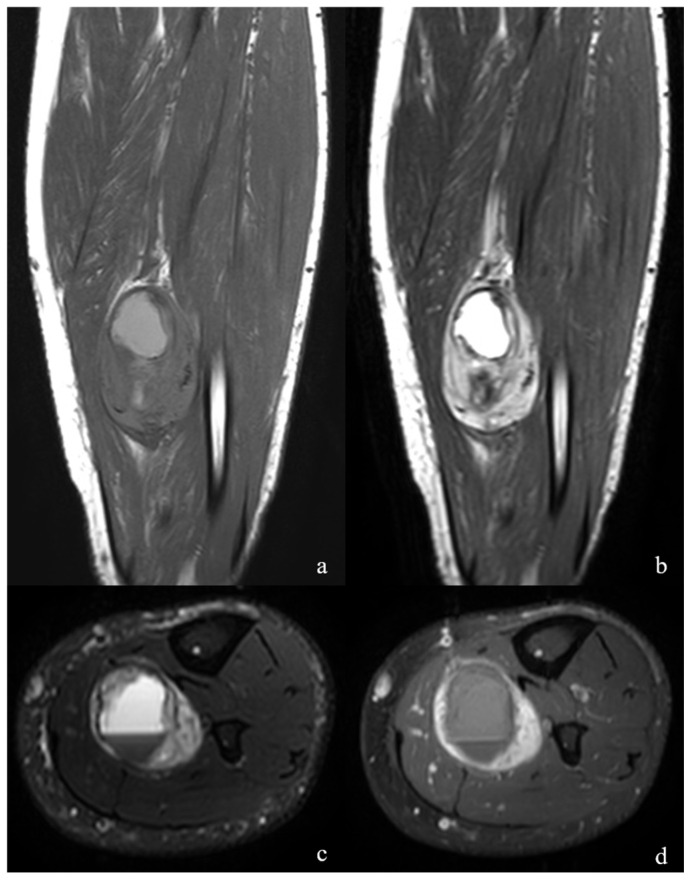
(a). The coronal T1-weighted images of a 50-year-old man revealed a well-shaped mass within the muscle planes of the deep posterior compartment at the mid-crural level, exhibiting an isointense signal peripherally and hyperintense hemorrhagic areas centrally. (b,c). The central hemorrhagic cystic component was clearly delineated on coronal T2-weighted and axial fat-saturated T2-weighted images. (d). Postcontrast axial T1-weighted sequences demonstrated marked enhancement in the peripheral solid portion of the lesion.
